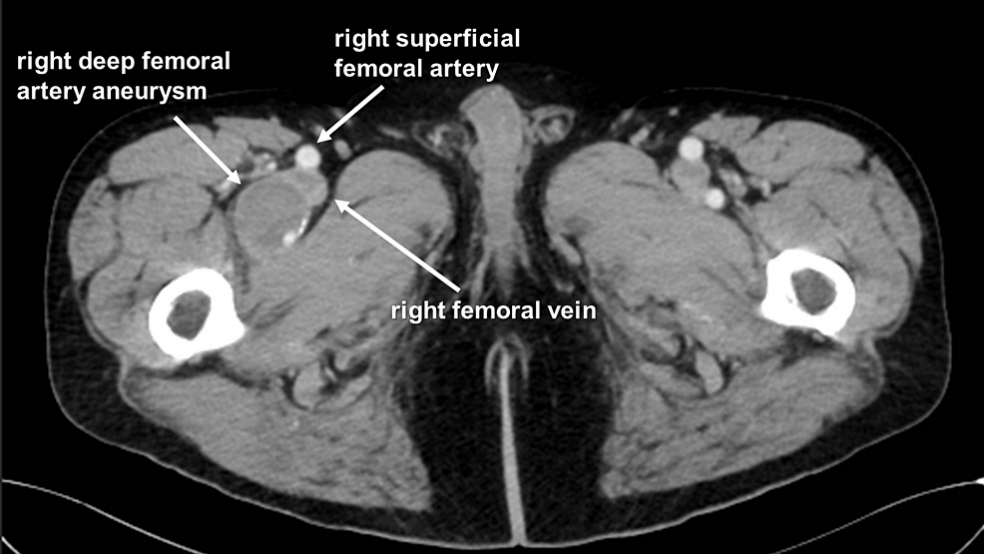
Preoperative contrast-enhanced CT Contrast-enhanced CT revealing right deep femoral artery aneurysm CT: computed tomography
